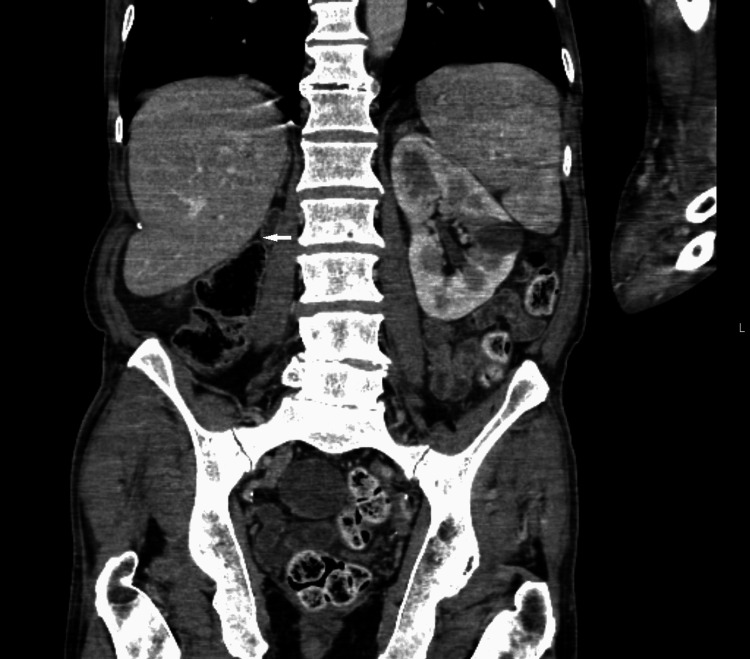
CT Abdomen (Coronal View): Absent right kidney, dilated seminal vesicle, and no locoregional lymphadenopathy
